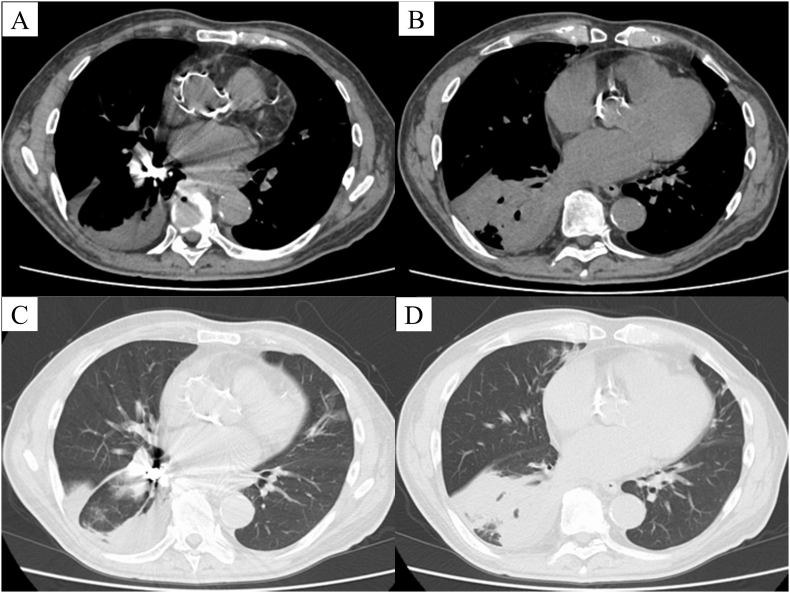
Thoracic computed tomographic (CT) images are shown. (A) Thoracic CT revealing a metallic foreign body, with artifacts in the right basal bronchus; (B–D) in addition to infiltration and consolidation in the right lower lobe and a small pleural effusion.
